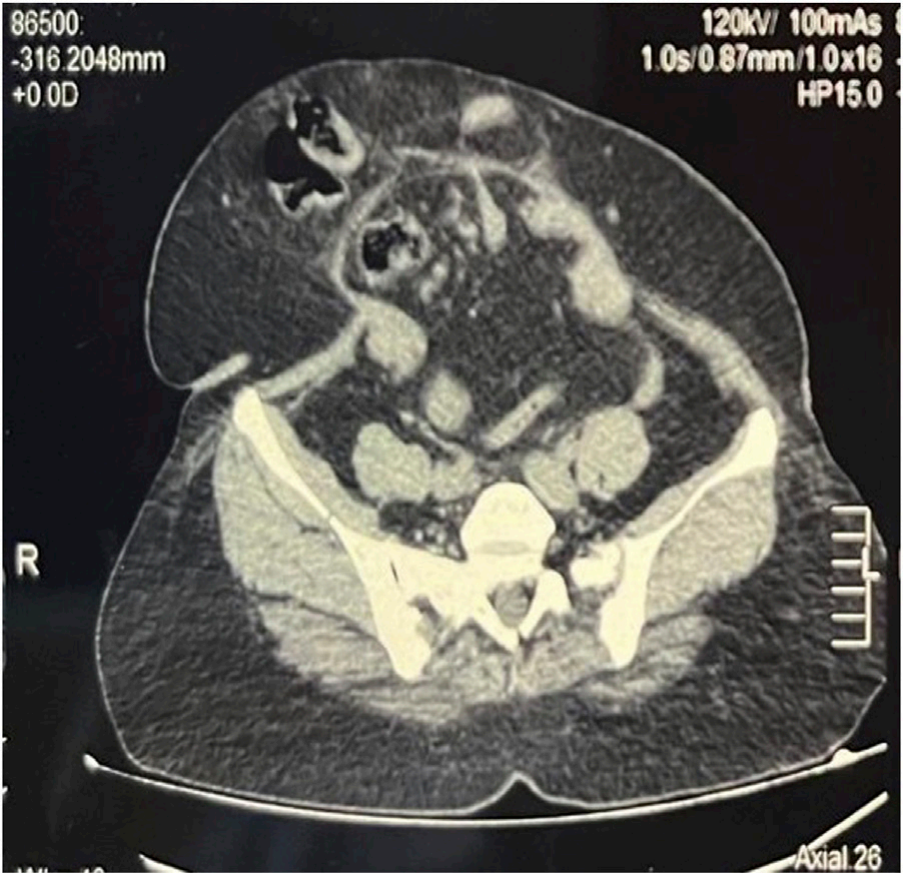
Post BT CT Abdomen showing relaxed and elongated abdominal wall muscles, with rectus moving closer to the midline.
